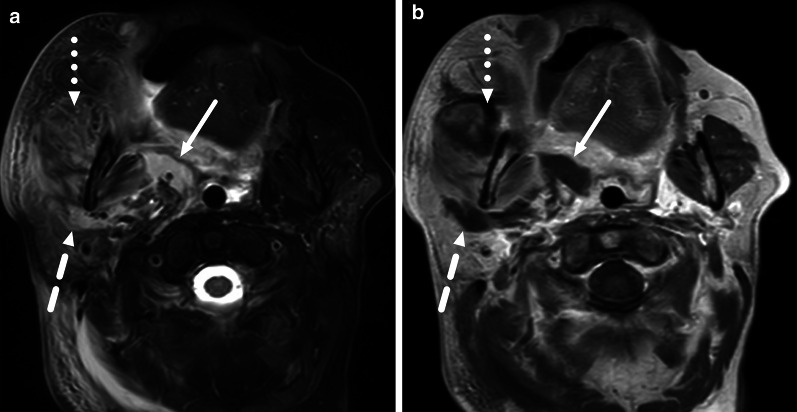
Multi-space abscess on axial fat-suppressed T2-weighted (a) and post-contrast T1-weighted (b) images. An odontogenic abscess affects the parapharyngeal (arrows), parotid (dashed arrows), and masticator (dotted arrows) spaces
